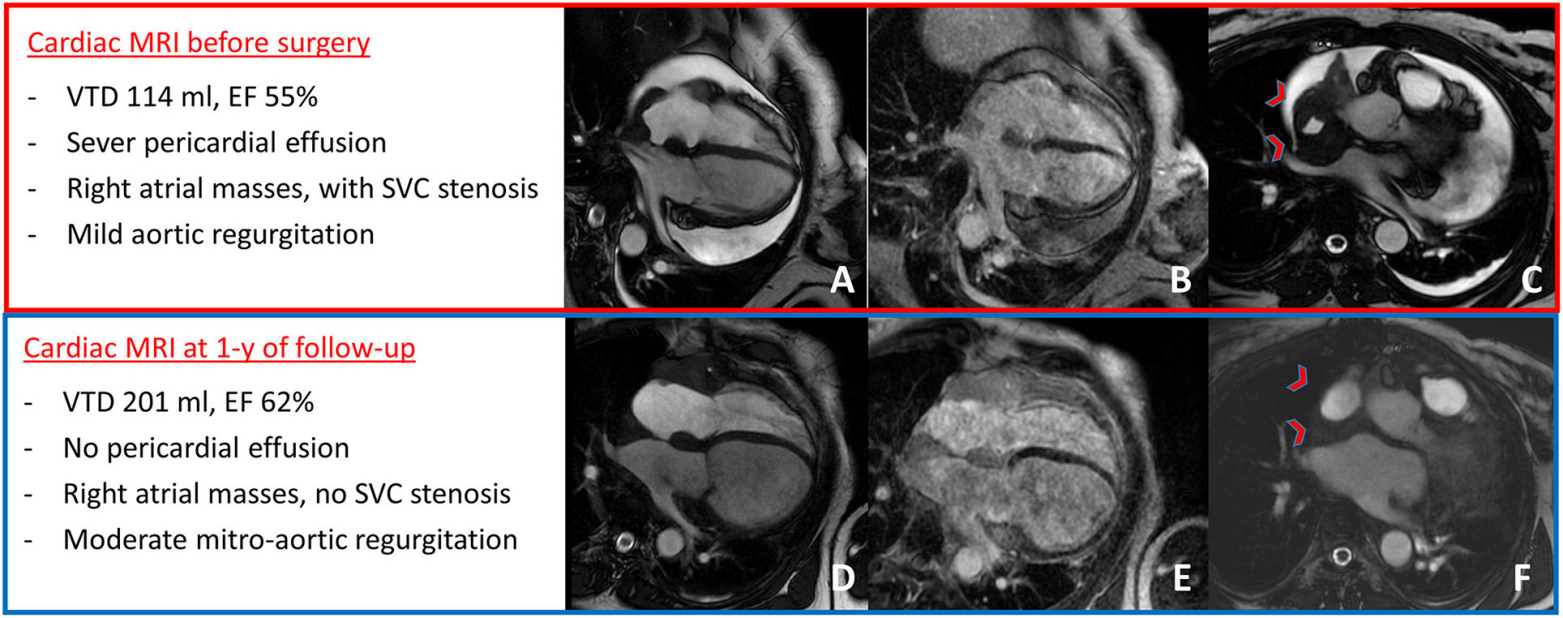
Cardiac MRI at presentation showed severe pericardial effusion with mild aortic regurgitation (A), right atrial masses with LGE (B), and mass infiltration of the superior cava vein causing stenosis [red arrow-heads in (C)]. After 1 year of follow-up, cardiac MRI showed no pericardial effusion with moderate mitro-aortic regurgitation (D) and mild increase in right atrial masses' size (E), but with no superior cava vein stenosis [red arrow-heads in (F)].
